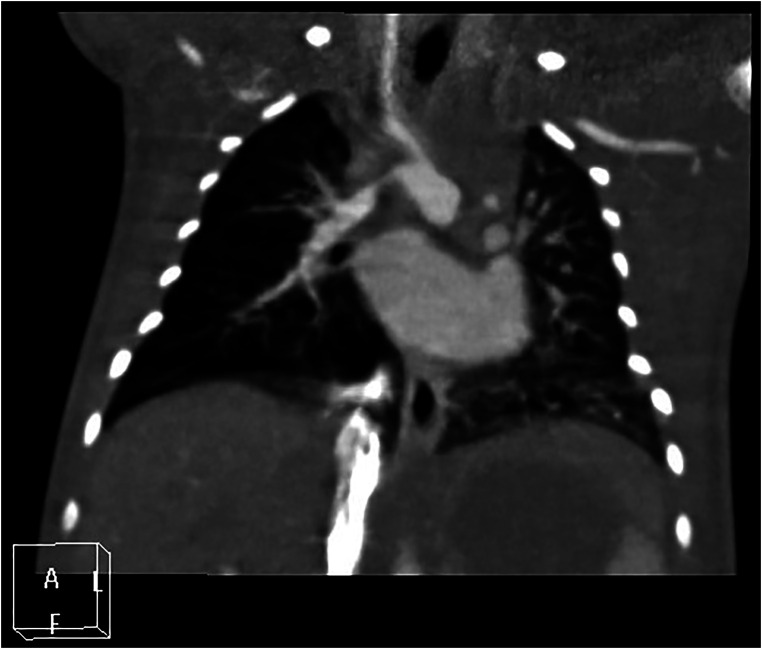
Right proximal interruption of the pulmonary artery, with left-sided aortic arch and right-sided patent ductus arteriosus in a 4-day-old female patient. A coronal view of the chest computed tomography angiography shows that the proximal right pulmonary artery is absent, and an open ductus arteriosus connects the proximal brachiocephalic trunk to the distal right pulmonary artery.
